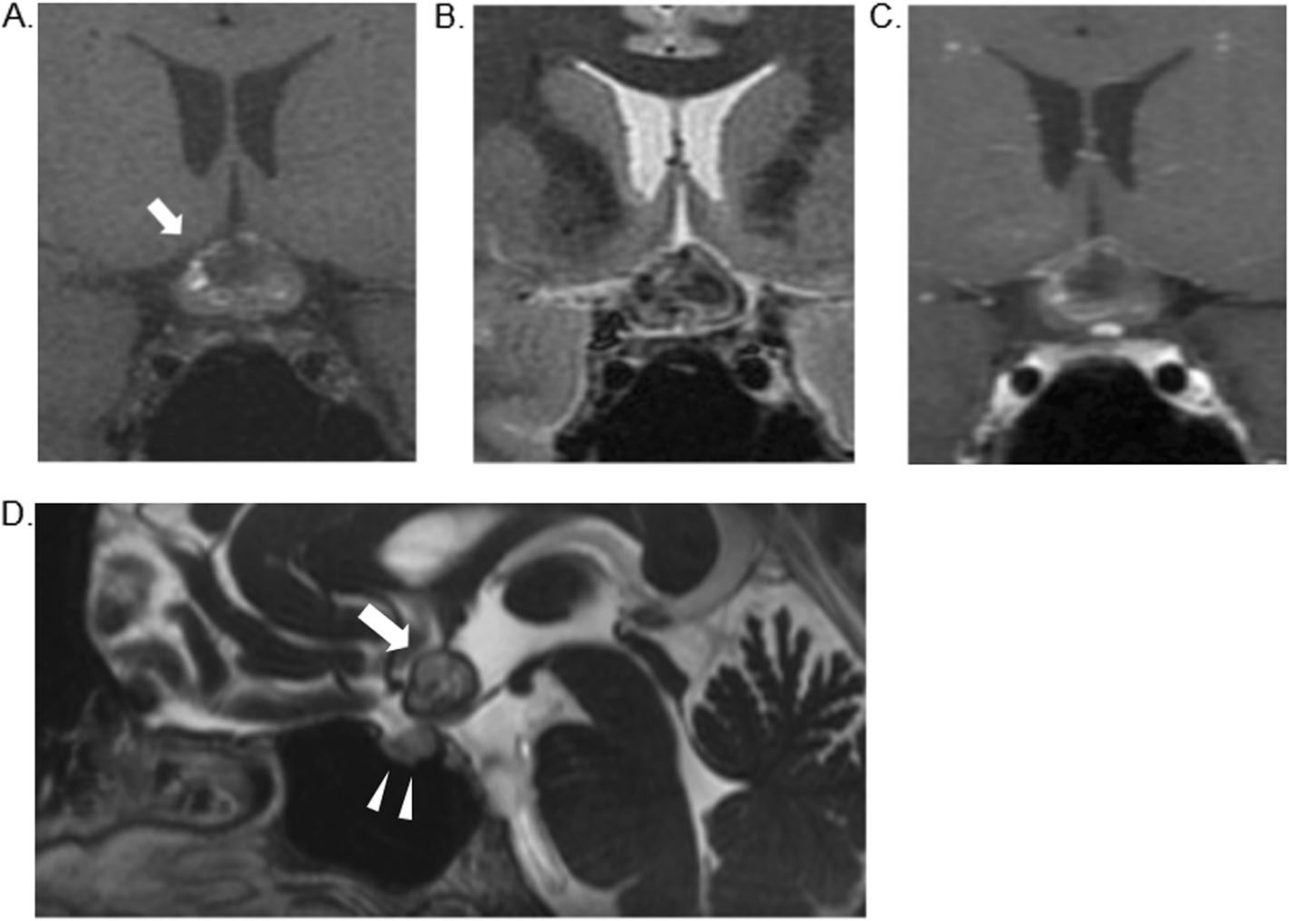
Coronal T1 weighted MRI showing a lesion with mixed hypo- and hyperintensity (white arrow) (a). Coronal T2 weighted MRI (b). Coronal post-gadolinium contrast-enhanced MRI showing minimal enhancement around the lesion (c). Sagittal MRI with contrast-enhanced constructive interference in steady state revealed the lesion (white arrow; major axis: 12.8 mm) (d). White arrow heads indicate the sella
